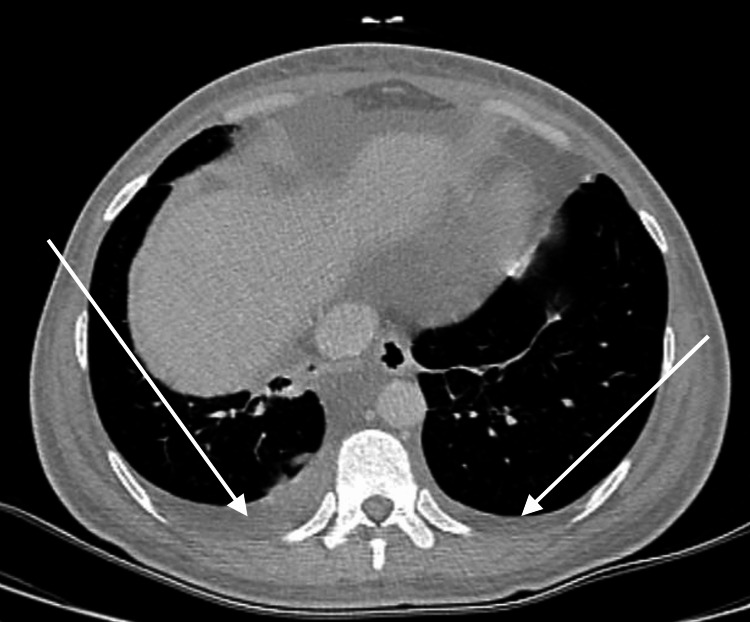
Patient's Thoracic CT Scan Revealing Bilateral Moderate Pleural Effusions (Arrows)
